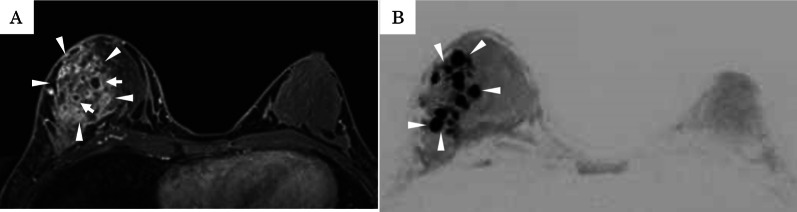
Dynamic contrast-enhanced magnetic resonance imaging: coronal view of the right breast. A T1-weighted image shows a 64 × 58 × 62 mm ill-defined high signal intensity lesion (arrowhead) with multiple ring enhancements in the upper half of the right breast (arrow). B Diffusion-weighted imaging shows strong diffusion restriction (arrowhead)
